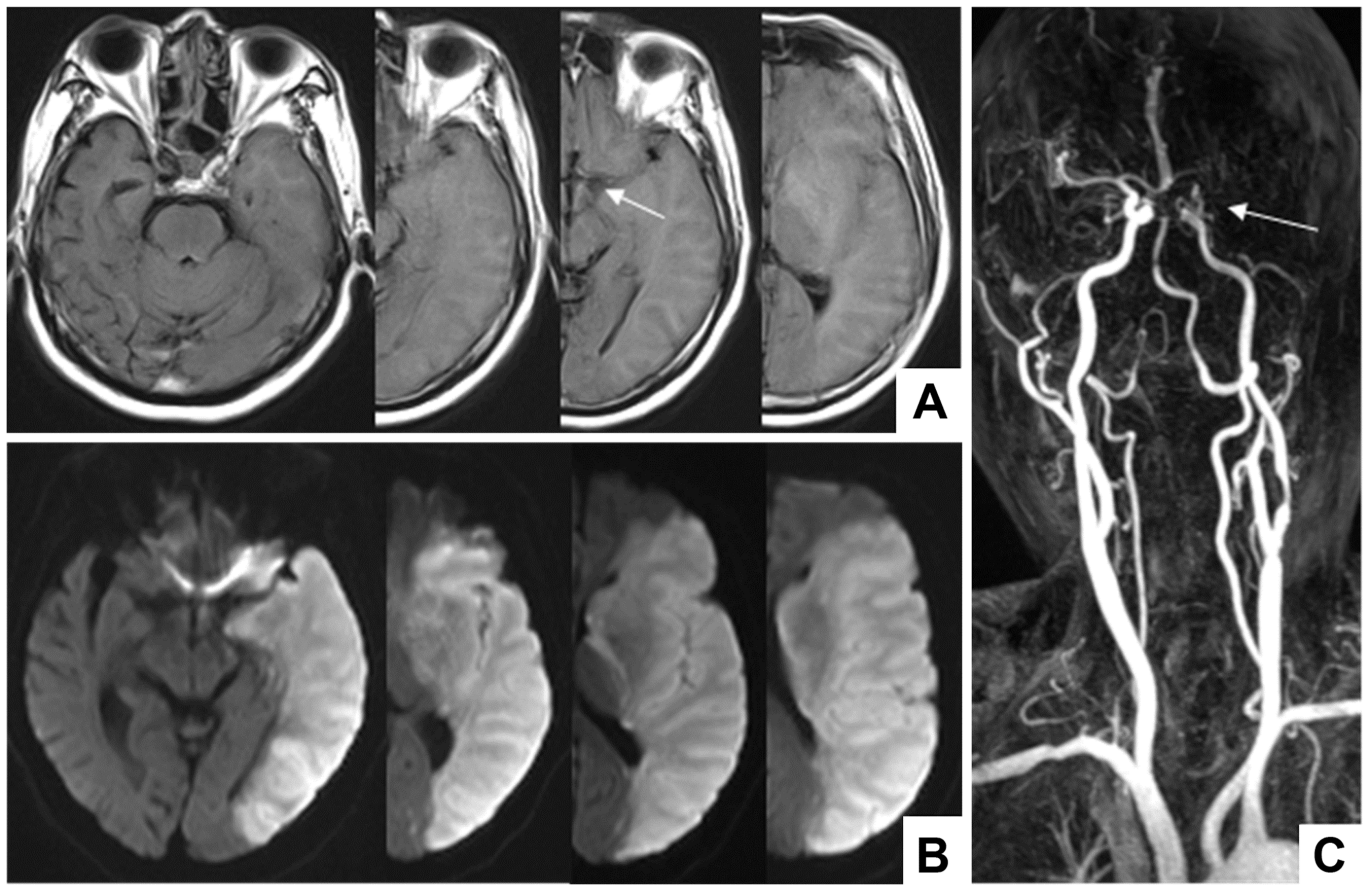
A patient with carotid artery occlusion. A: FVH was found in one image of the FLAIR sequence, that was located at the proximal segment; therefore, this patient was defined in the Low FVHs patients and Proximal FVH patients respectively; B: DWI sequence indicated a massive cerebral infarction of the left hemisphere; C: carotid MRA showed distal occlusion of the left internal carotid artery.
